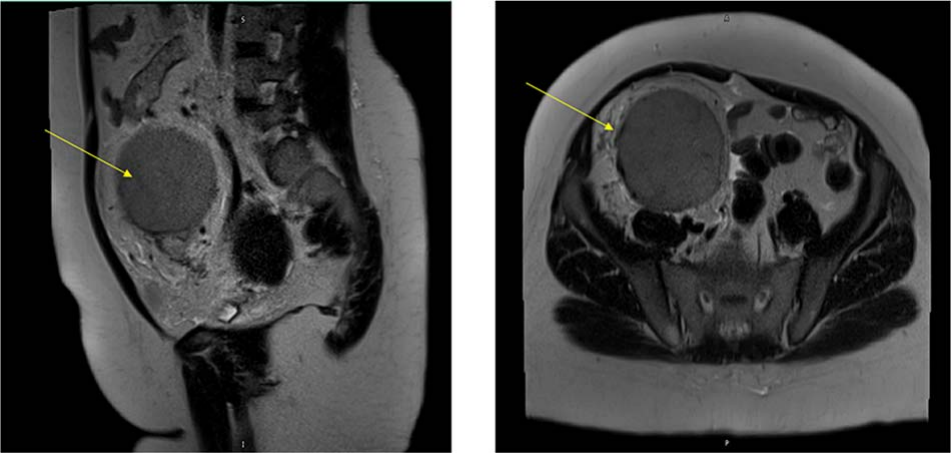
MRI abdomen signifying the mass and its location, extent in both views (Arrow points at the mass).
